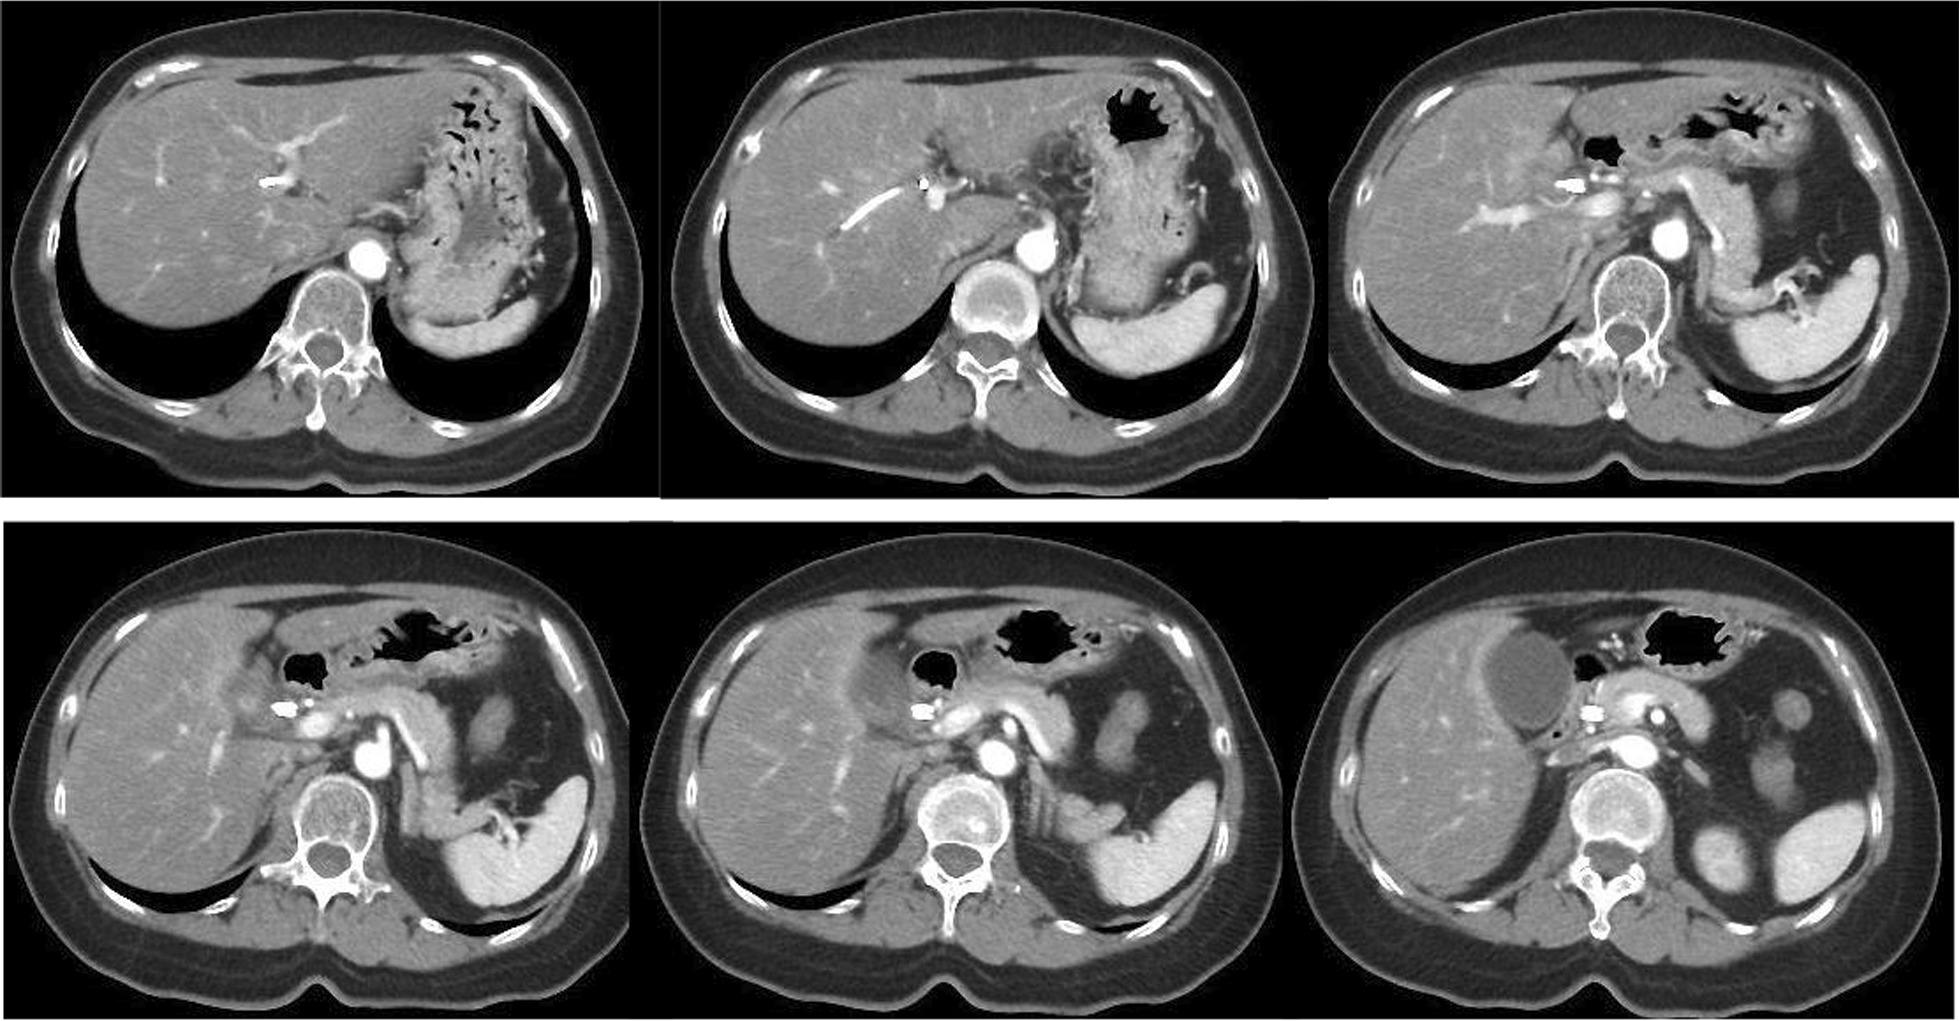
After six courses of chemotherapy, computed tomography examination showed that the mass in the neck of the gallbladder had shrunk to 3 cm, and endoscopic retrograde cholangiopancreatography showed improvement of hilar duct stenosis.
